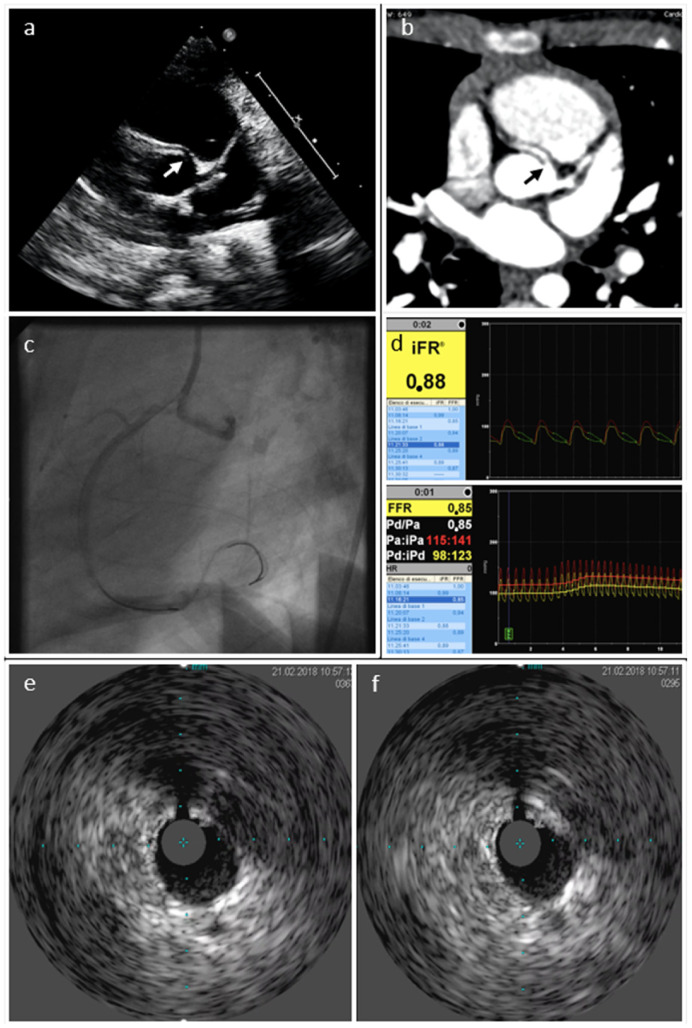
A 7-year-old patient with chest pain. Ultrasound examination showed a right coronary artery from the left sinus (a), confirmed by computed tomography ((b) arrow); coronary angiography (c) showed an iFR in the grey zone (d); intravascular ultrasound showed a ring of tissue around the ostium of the right coronary artery with systolic contraction, attributable to intramural course (e,f).
